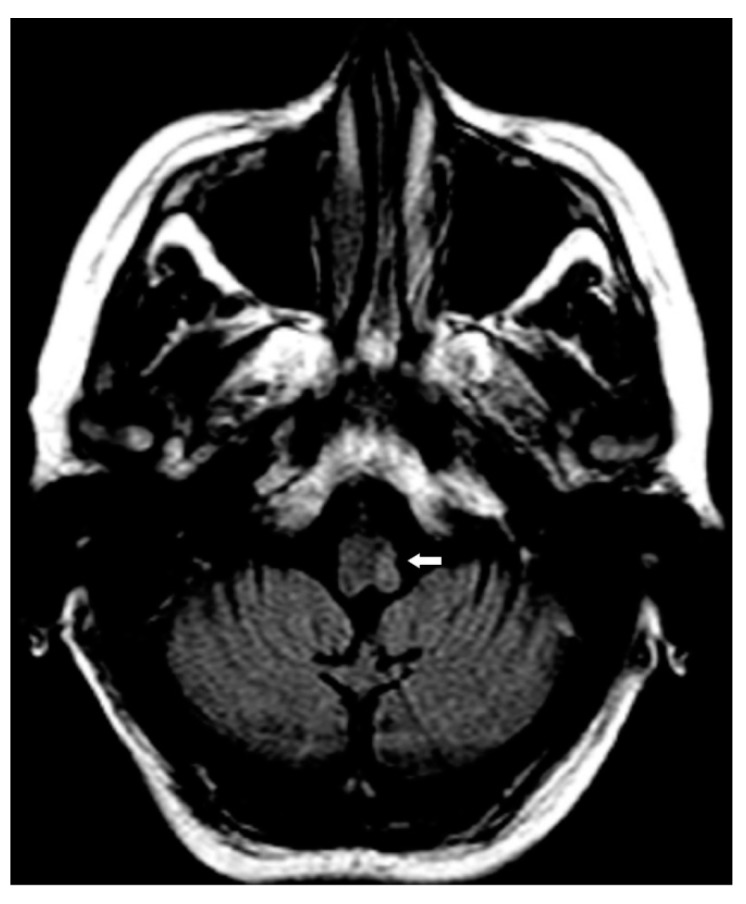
An axial FLAIR-weighted MRI image showing a hyperintense area (white arrow) in the left lateral medulla, suggestive of infarct.
